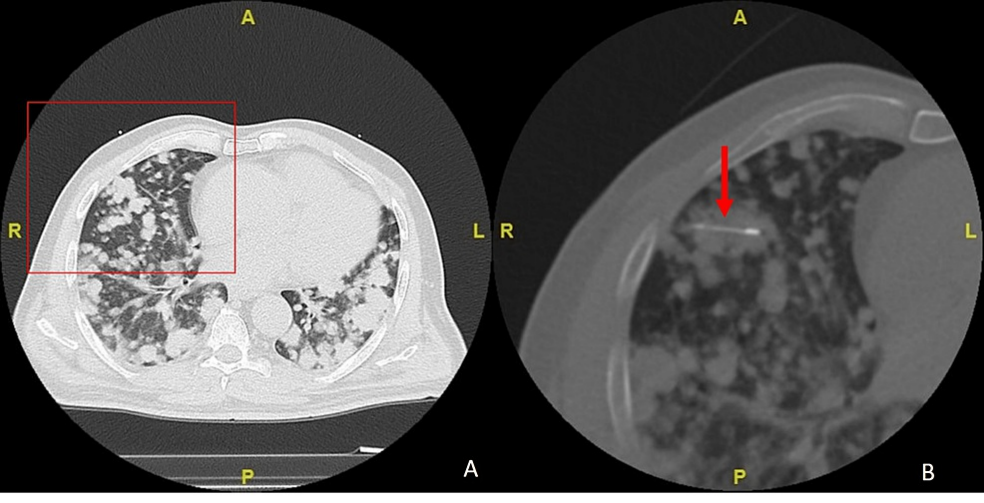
Axial Computed Tomography (CT) in lung window demonstrating multiple lung nodules (A). Magnified local view (B) of the segment framed red in (A) for accurate needle placement with a 15mm core biopsy needle (red arrow) within one of the largest cluster of nodules located peripherally in the right lower lobe.
